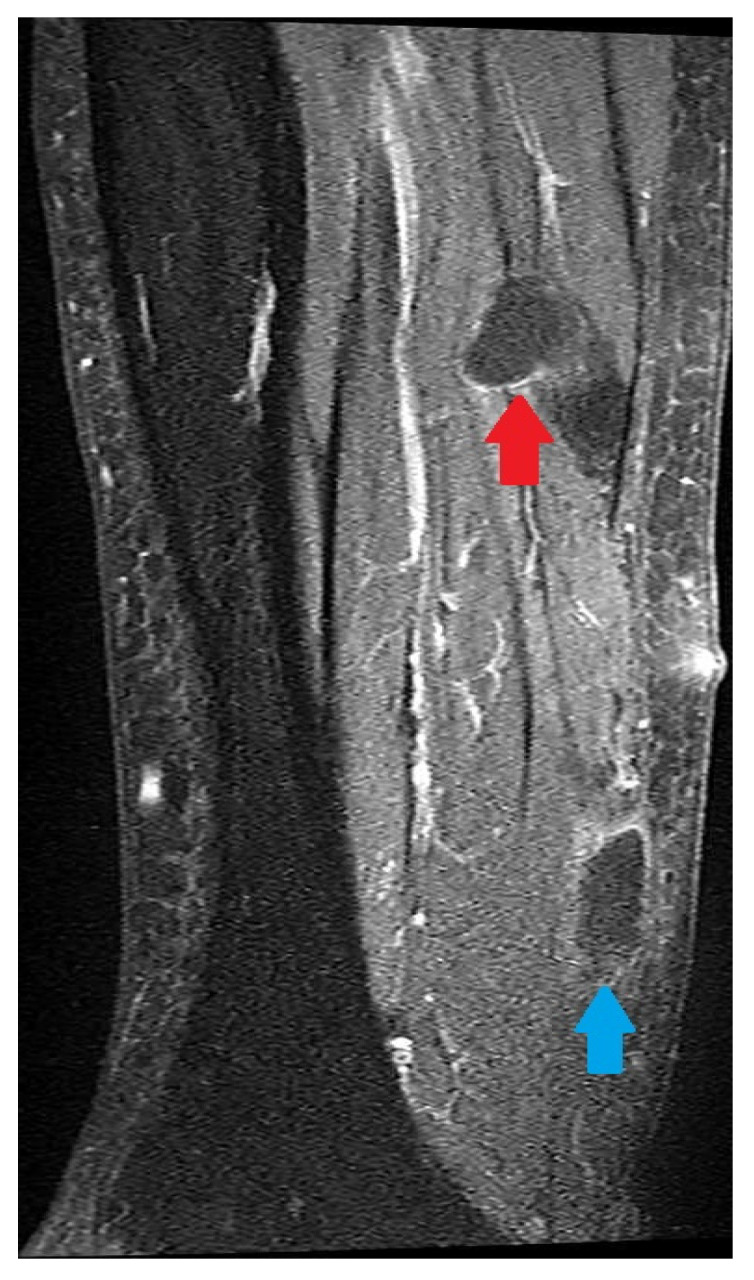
A postcontrast magnetic resonance imaging (MRI) scan of the patient’s left calf, performed on 18 June 2024, after surgical incision, revealed two focal lesions with different morphological features (red and blue arrows). The first one was situated in the proximal part of the calf, inside the soleus muscle. At the postcontrast T1 WI MRI scan, the lesion was dominantly hypointensive compared with the surrounding muscle with discreet postcontrast ring enhancement, with defined contours (red arrow). The second lesion was an extramuscular, subfascial collection of dense fluid, located in the distal third of the left calf, near the dorsal part of the soleus muscle and the dorsal part of the Achilles tendon. On a postcontrast T1 WI MRI scan, the lesion was hypointense with postcontrast ring enhancement and no clear border (blue arrow).
